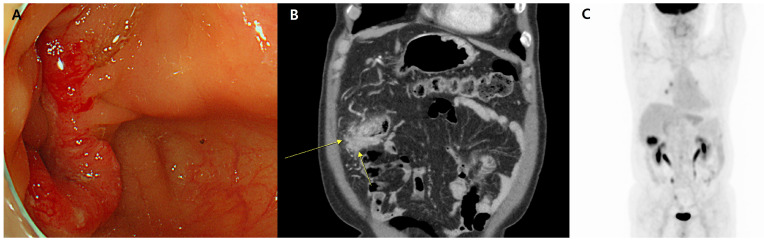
Endoscopic and radiologic images. (A) Endoscopic image revealing a prominent mass lesion in the transverse colon, characterized by mucosal irregularity and friability. (B) Axial T2-weighted magnetic resonance imaging (MRI) depicting the transverse colon that presents irregular concentric wall thickening with stenosis at the proximal transverse colon near the hepatic flexure, extending approximately 6 cm (arrows). These findings suggest transverse colon cancer (cT4aN2Mx) and demonstrate multifocal extramural venous invasions as well as pericolic nodular extensions, serosal thickening with enhancement, and omental infiltration. (C) Positron emission tomography-computed tomography (PET-CT), which revealed hypermetabolic wall thickening in the T-colon with pericolic infiltration and subtle hypermetabolic pericolic lymph nodes. No other organs were involved except for the colon.
